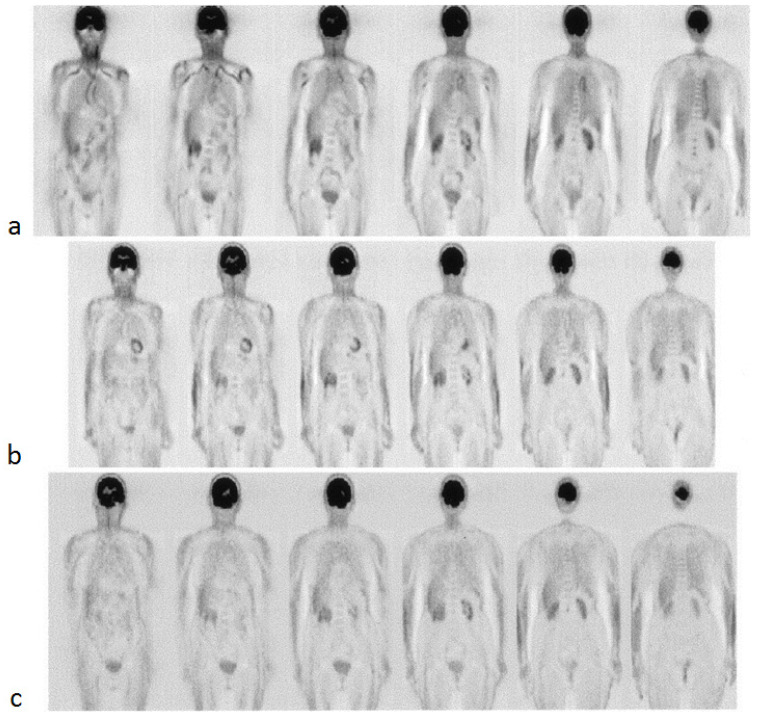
FDG-PET images of a 78-year-old woman with giant cell arteritis at the baseline (a), 3 months of therapy (b), and 6 months of therapy (c). High-tracer uptake is initially present and visible in the thoracic aorta and subclavian arteries, as evident in the first two images from the left (a), which progressively decreased after treatment with steroids (from Blockmans et al. [142] with permission).
